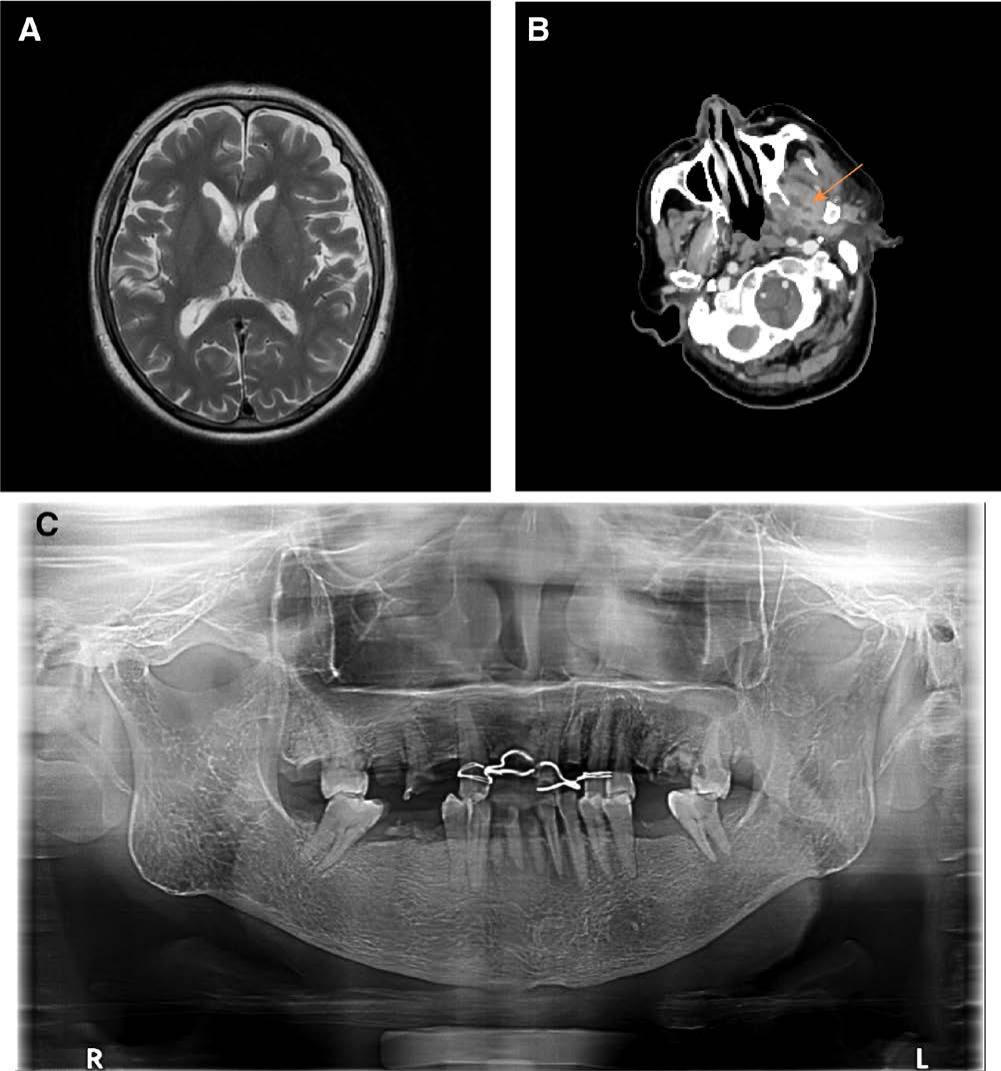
Imaging and clinical findings of case 2. (A) Cranial MRI on admission. (B) Extrapterygoid swelling with multiple small hypodense shadows (arrow). (C) Intraoral dental condition. MRI = magnetic resonance imaging.
